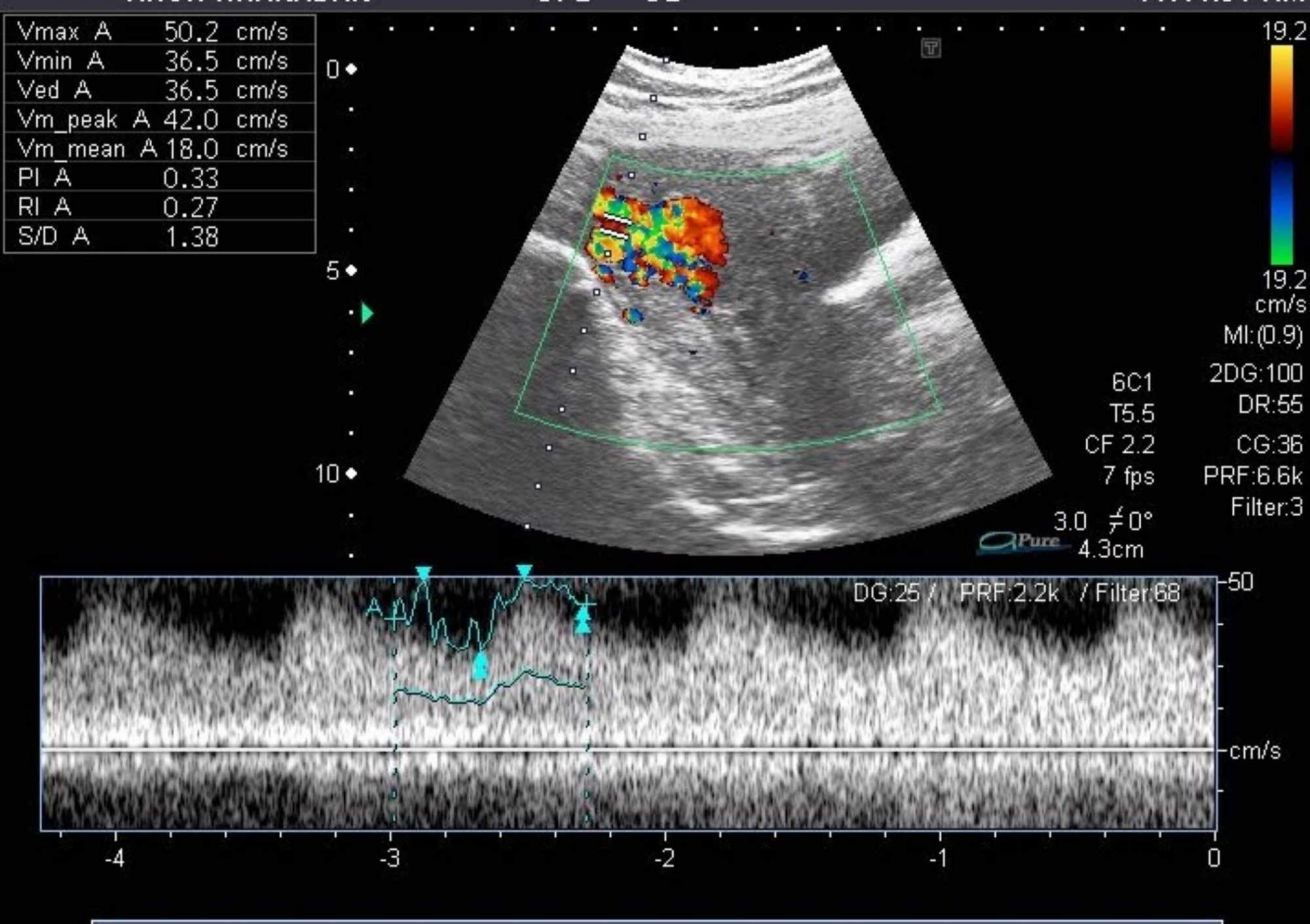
Doppler ultrasound showing increased vascularity in the endometrium
